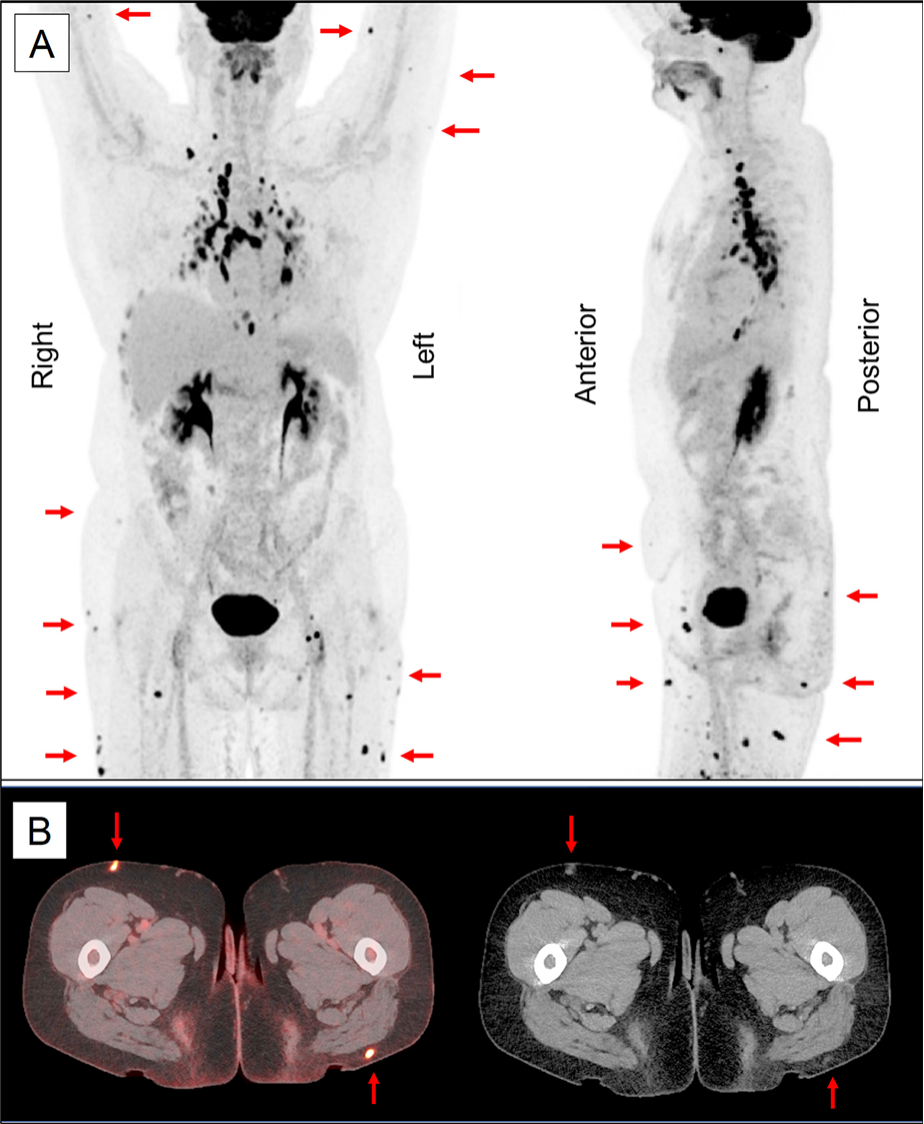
Several abnormal subcutaneous activities (indicated by red arrows) are seen in the latest ^18^F-Fluorodeoxyglucose (^18^F-FDG) positron emission tomography study. Corresponding hyperdense subcutaneous nodules on computer tomography (B). Additionally, the patient exhibited lymph node activation in the mediastinum and mild activity in the myocardium. Notably, the ^18^F-FDG activities observed next to the liver were attributed to rib fractures (as seen in A)
